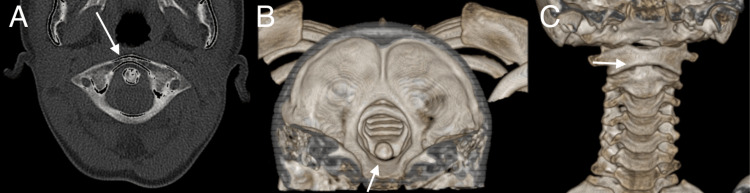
Repeated (A) axial cut of the CT scan and (B) axial and (C) coronal view of the 3D reconstruction CT scan of the cervical spine seven months post-removal of the halo vest showing no recurrence (arrows). CT: computed tomography
